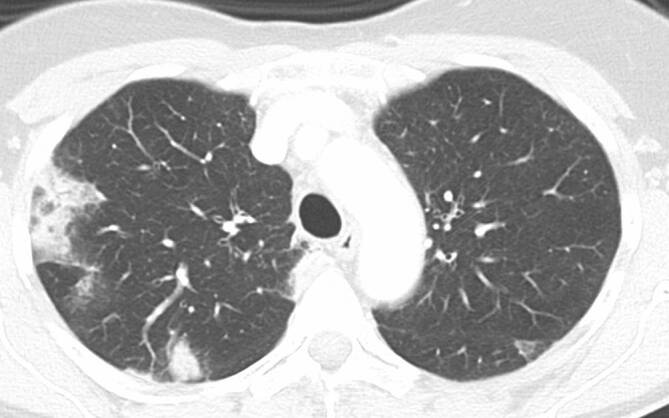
Das kardiogene Lungenödem, verursacht durch eine Linksherzinsuffizienz, zeigt sich in der CT mit verdickten interlobulären Septen und Bronchialwänden als Zeichen eines interstitiellen Lungenödems. Des Weiteren finden sich symmetrische, teils multiple oder diffuse Milchglasverdichtungen und Konsolidierungen als radiologische Manifestationen eines alveolären Lungenödems [44]. Zusätzlich sind ein vergrößertes Herz und bilaterale Pleuraergüsse hinweisend auf ein kardiogenes Lungenödem [44].
